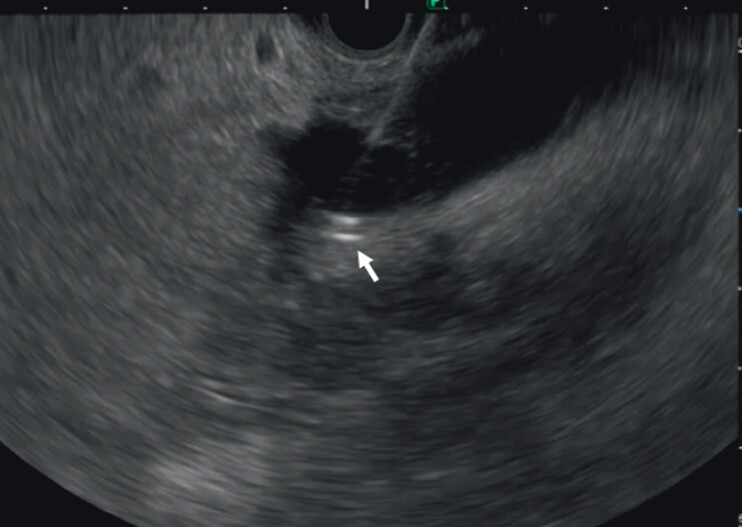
Endoscopic ultrasonography image showing the migrated stent.
